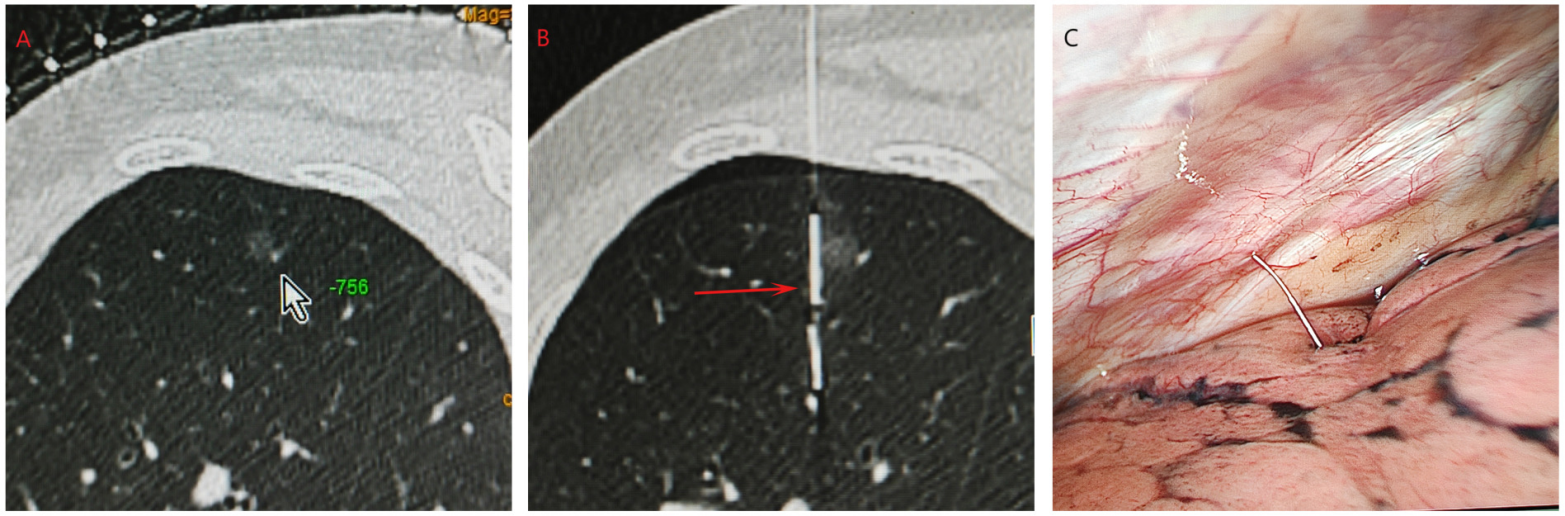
The procedures of CT-guided accura wire localization. (a) A GGN located at left upper lobe (arrow). (b) The Accura wire (red arrow) was placed near the GGN. (c) The Accura wire could be visualized during the VATS.
